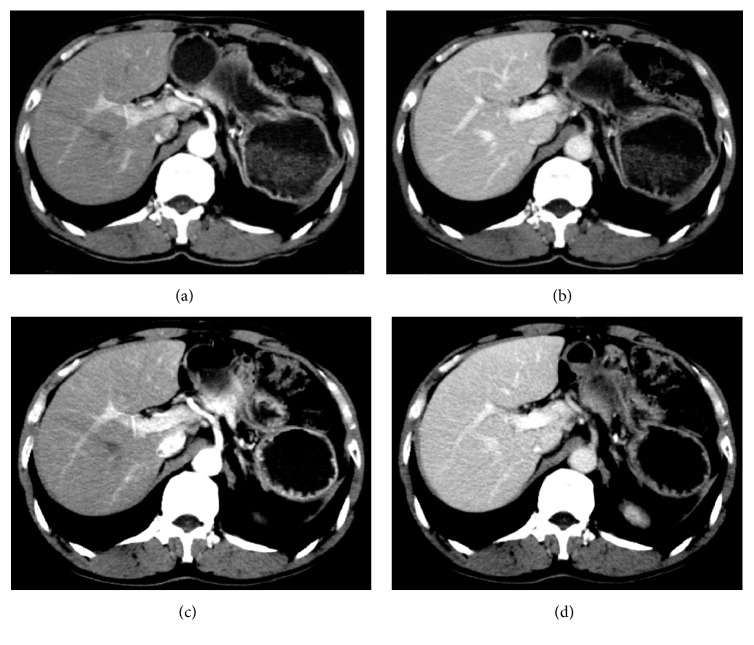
The follow-up CT image pairs of a 63-year-old male patient with a body weight of 47 kg after insulin tumor resection. Images (a) and (b) were scanned with injection protocol in G1 (contrast medium dose: 78 ml). The liver parenchyma CT values during HAP and PVP, portal vein CT value during PVP, and liver parenchyma ΔHU during HAP and PVP were 75 HU, 106 UH, 136 HU, 35 HU, and 66 HU, respectively. Images (c) and (d) were scanned with injection protocol in G2 (contrast medium dose: 87 ml). The liver parenchyma CT values during HAP and PVP, portal vein CT value during PVP, and liver parenchyma ΔHU during HAP and PVP were 77 HU, 115 HU, 153 HU, 37 HU, and 75 HU, respectively. The liver anatomical structures of the two pairs of images were both visualized clearly.
